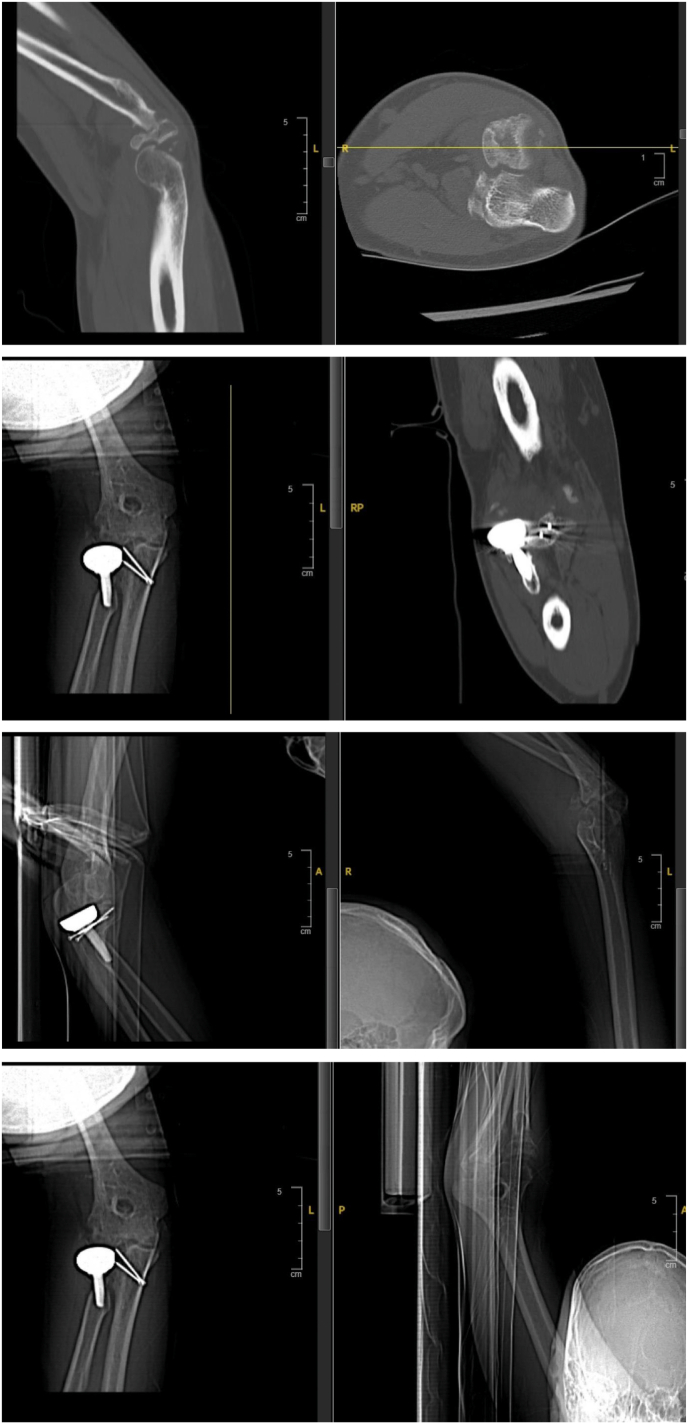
A comparison between elbow CT scan showed pre operative injuries and post operative fixation in axial and sagittal views.
